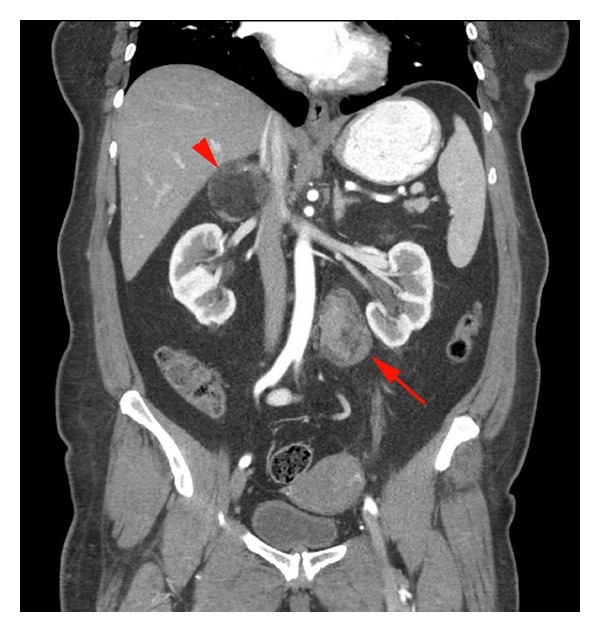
Coronal image from contrast enhanced CT of the abdomen/pelvis. A heterogeneous soft tissue mass is seen in the left para-aortic retroperitoneum, inferior to the renal hilum (arrow). A predominantly fat containing right adrenal mass is also noted with punctate calcifications (arrowhead). The left adrenal gland is normal.
